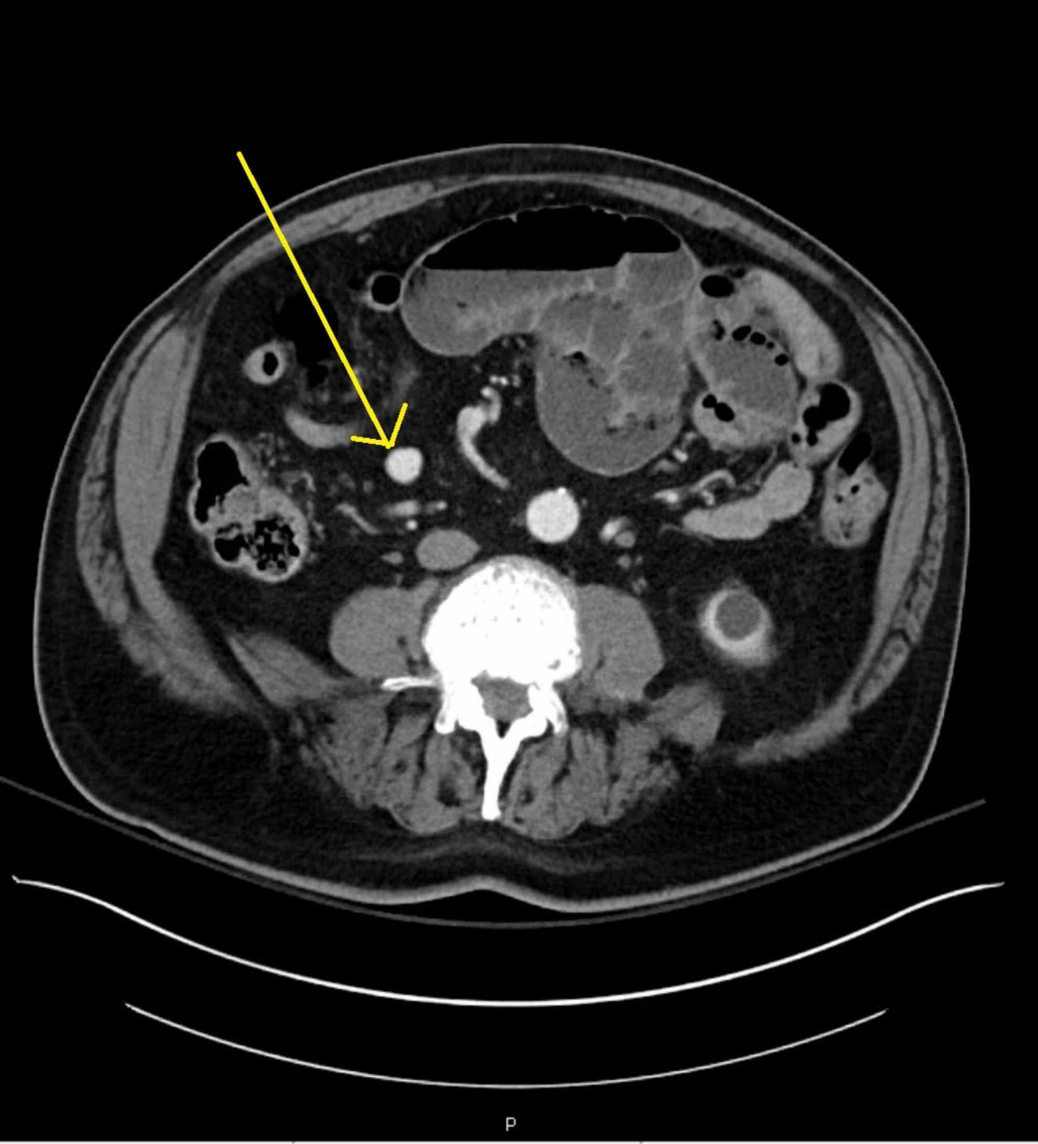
Pseudoaneurysm as seen on CT of the abdomen. Yellow arrow points to pseudoaneurysm.
